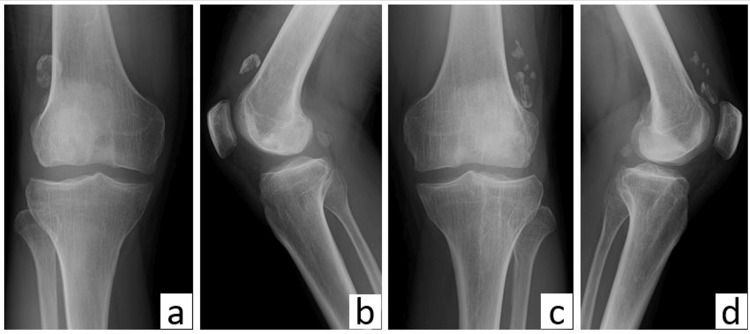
Plain radiographs of both knees prior to surgery (a) Anteroposterior and (b) lateral plain radiographs of the right knee; (c) anteroposterior and (d) lateral plain radiographs of the left knee.
